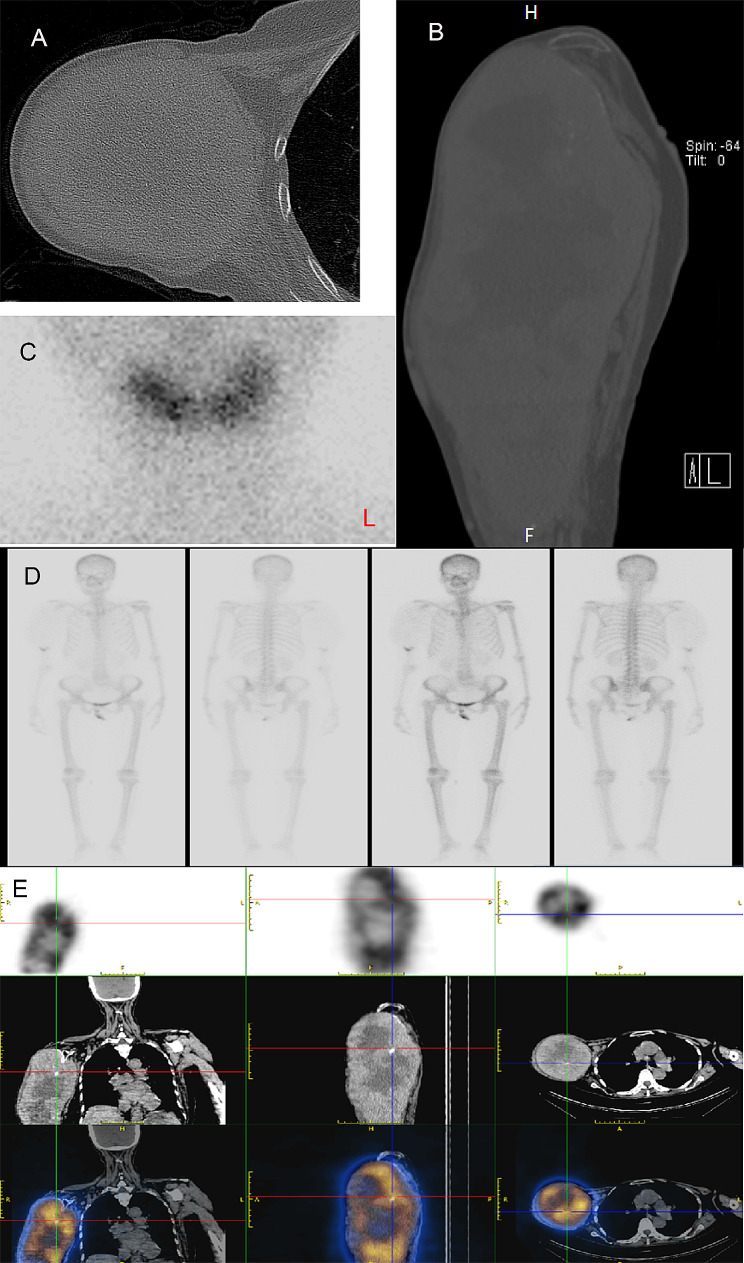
(A, B) Computed tomography (CT) images of the right shoulder and upper arm. (C) Thyroid emission computed tomography scan. (D) Whole-body bone imaging. (E) Whole-body imaging on the fifth day after oral administration of 350 mCi of 131I. There was no clear residual functional tissue in the thyroid region, but obvious functional metastasis could be seen in the right upper arm
